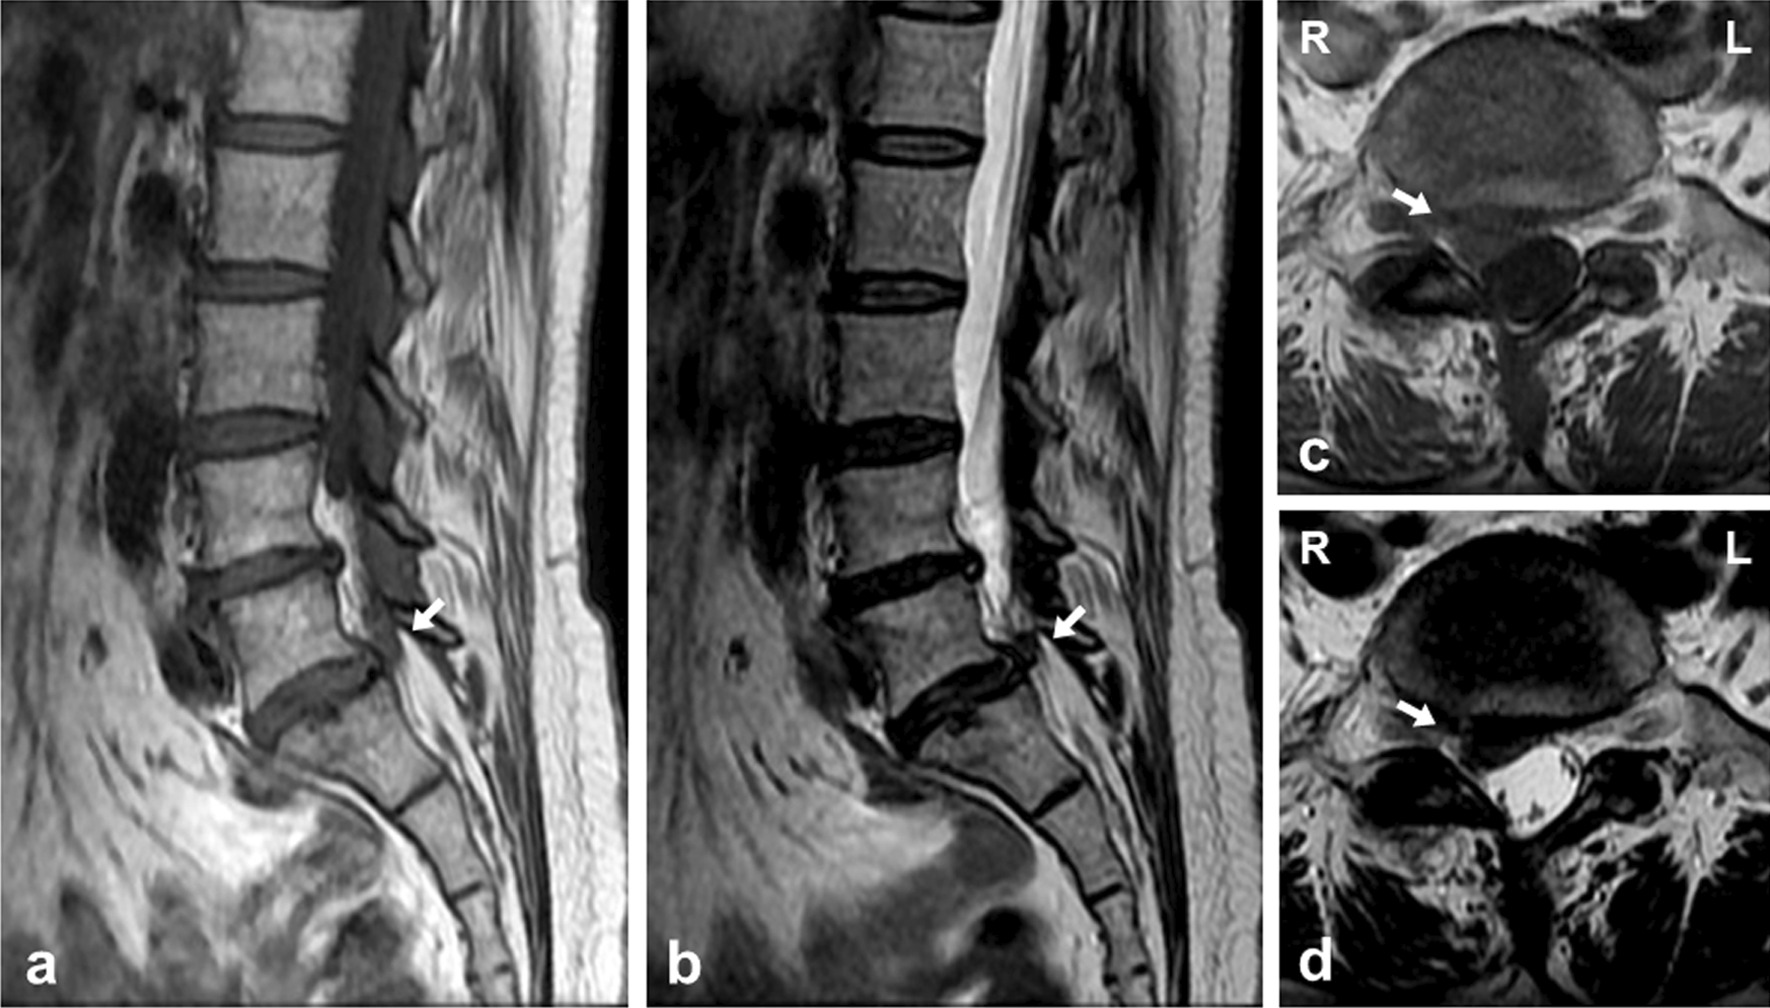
MRI. Herniated nucleus pulposus on the right side at the L5/S1 intervertebral disc level was revealed (white arrows). a T1 parasagittal view. b T2 parasagittal view. c T1 axial view. d T2 axial view
